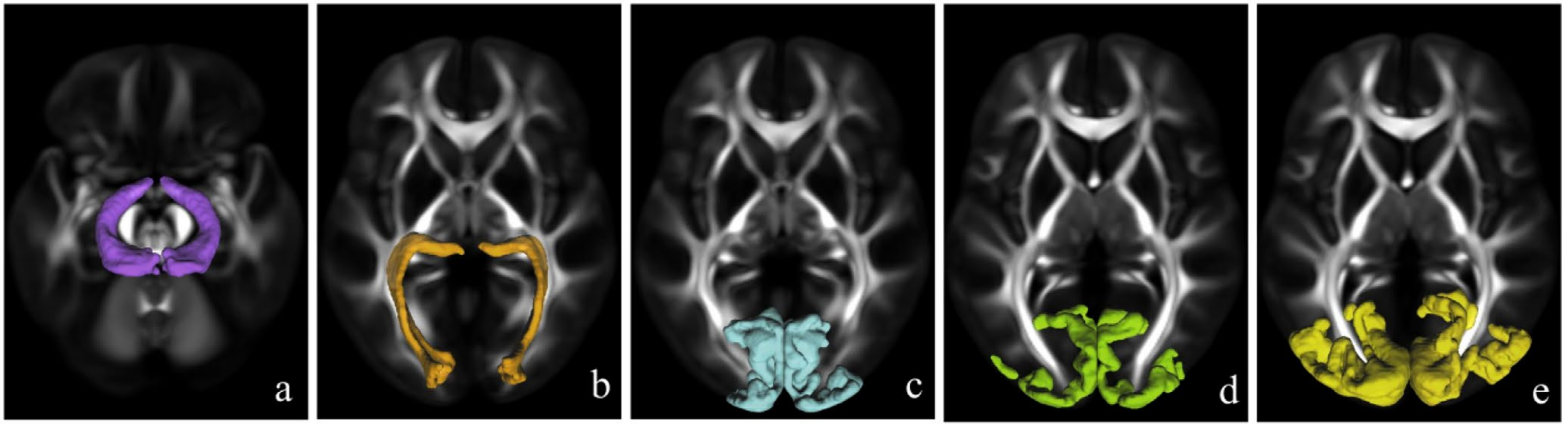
Template‐based masks of the intracranial visual pathway for MAP‐MRI parameter extraction. The mask of OT and OR derived from the HCP‐1065 diffusion template (a, b). The masks of BA 17, 18, and 19 were respectively extracted from the Brodmann area atlas (c–e). BA, Brodmann area; HCP, Human Connectome Project; MAP‐MRI, mean apparent propagator‐magnetic resonance imaging; OT, optic tract; OR, optic radiation.
